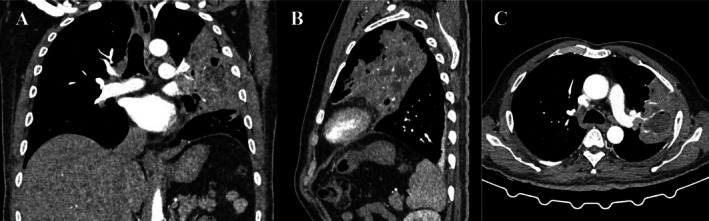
Computed tomography findings. Coronal (A), sagittal (B) and axial (C) planes. A second opinion contrast‐enhanced CT scan revealed an area of consolidation with multiple small cavities, necrotic component (24 HU) and contrast enhancement (79 HU) of the upper left lobe, indicating necrotizing pneumonia with mucous plaque.
